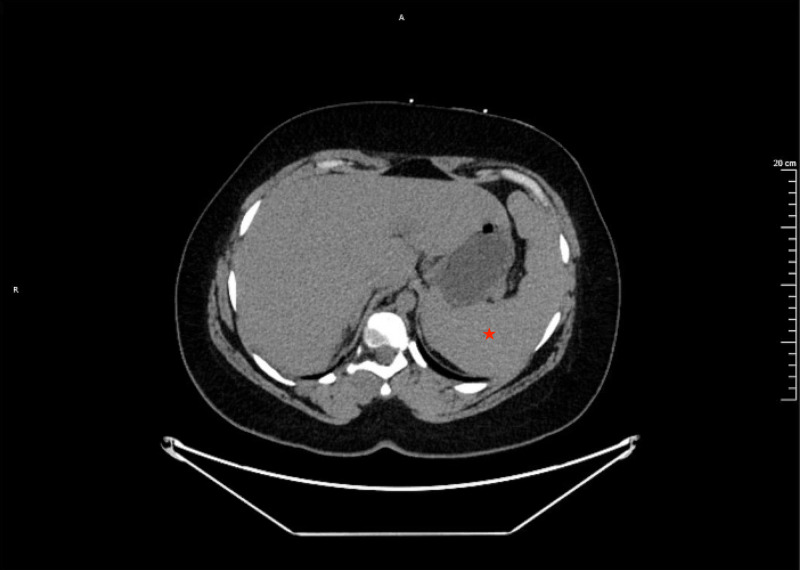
CT of the abdomen showing prominent splenomegaly measuring 15.9 cm (normal: ≤12 cm) along the anteroposterior diameter.
